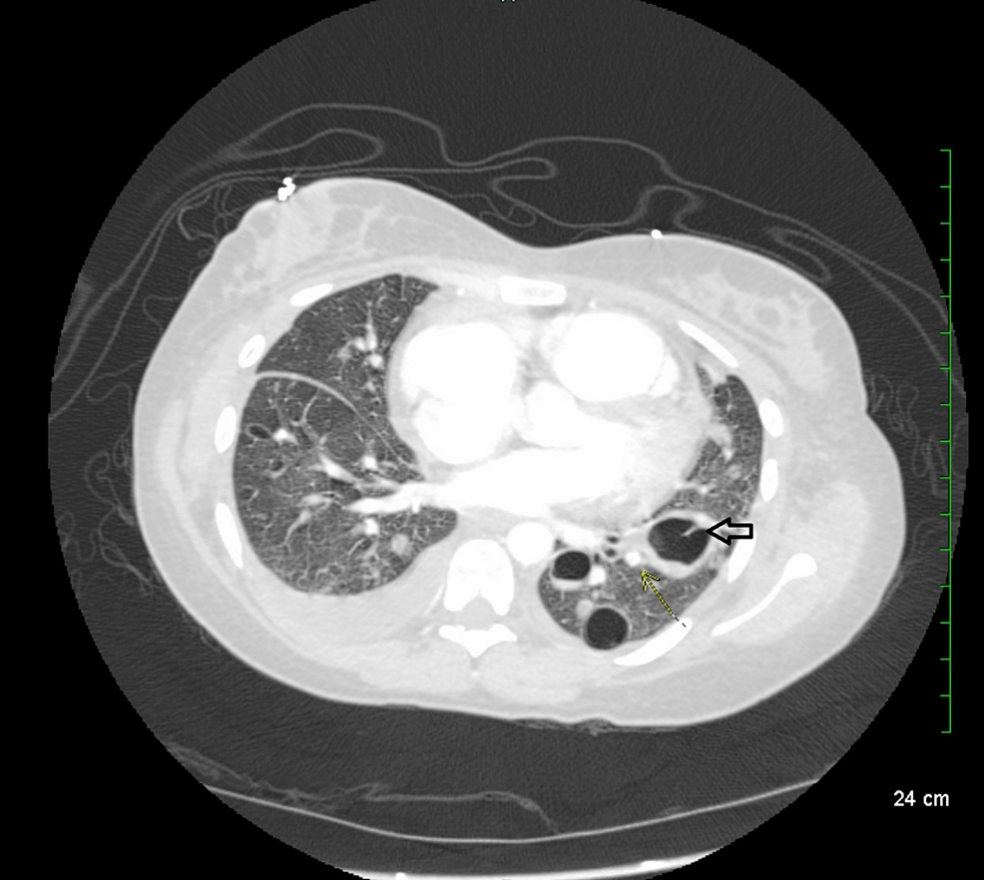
CT angiography chest on admission demonstrating multiple cavitary lesions, one of which is marked with a transparent back arrow.
